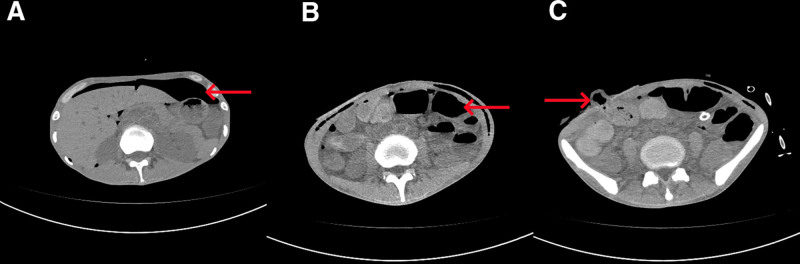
Computed tomography (CT) imaging of ileal perforation. The first pre-operation examination in the axial position revealed a large amount of free gas (A) and the second pre-operation and post-operation examination in the axial positions (B and C) revealed small bowel dilation, gas–liquid level, seroperitoneum, and the stoma location in the abdominal wall (indicated by arrows).
